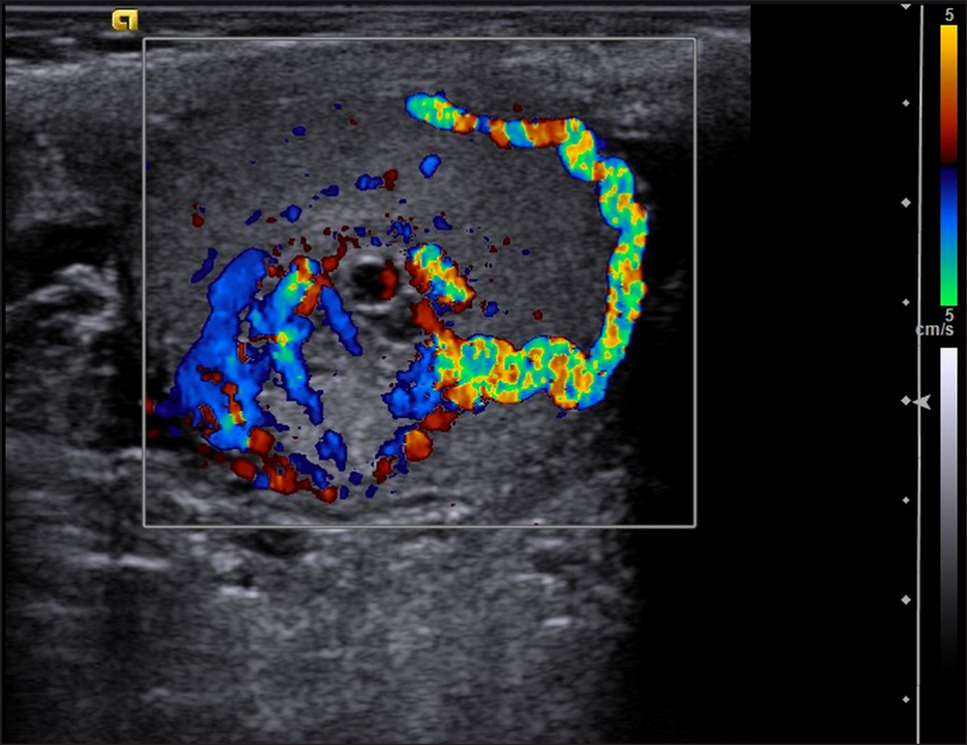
Colour Doppler ultrasound demonstrates tumoral hypervascularisation.
